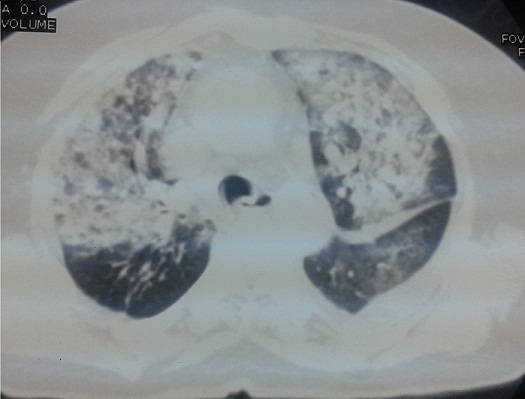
Computed tomography with enhancement showing patchy areas of ground-glass opacity in both lung fileds
